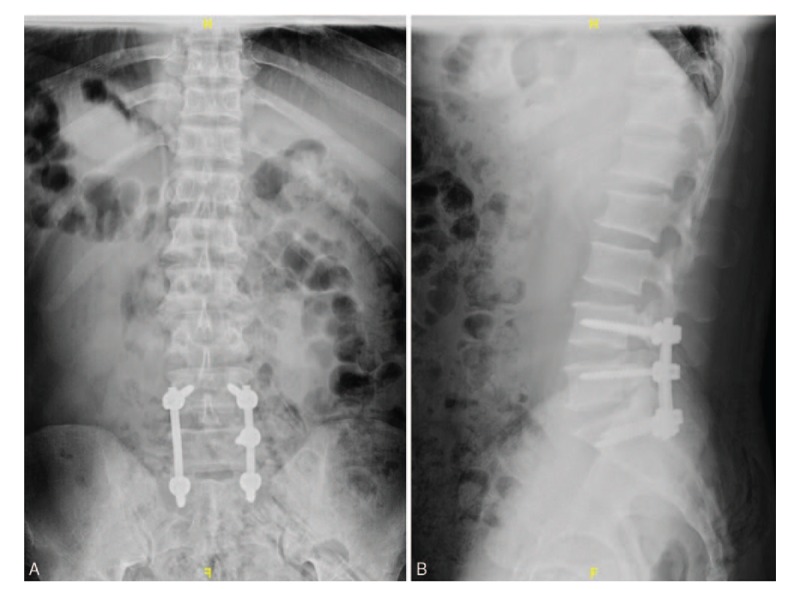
(A, B) Posteroanterior and lateral X-ray images of the lumbar spine obtained after the 1st operation.
